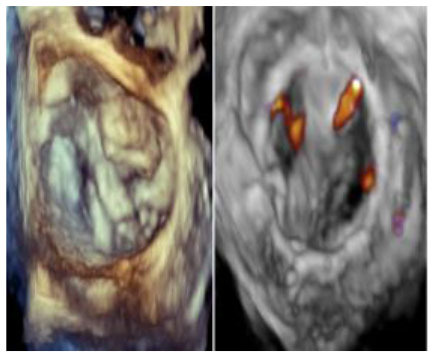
No left ventricular outflow tract obstructions were detected due to the presence or dislocation of the TAVI prosthesis (mean LVOT gradient of 8 ± 1 mmHg). We were able to fix a mild residual leak between the Sapien and the pericardial skirt in two out of five patients using intraoperative transesophageal echocardiographic control (3D zoom with color, “en face” or “surgical” projection of the MV).
